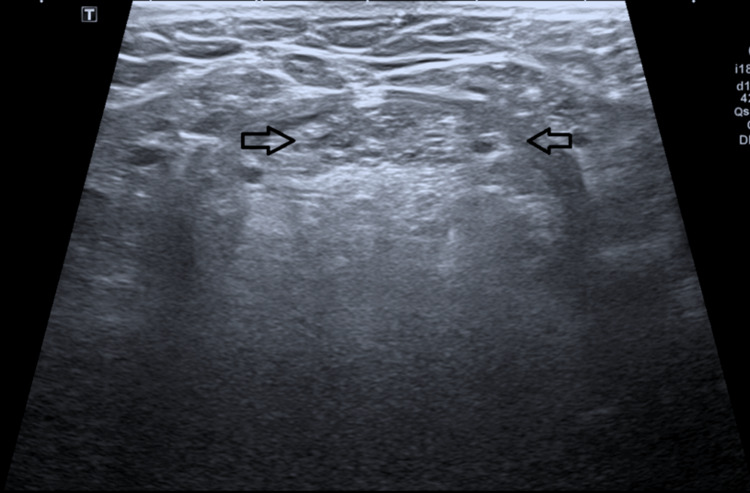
Ultrasound of the thyroid demonstrating mild enlargement, demonstrating inhomogeneous echo pattern with pseudonodular appearance/micronodules and mild increase in vascularity, with features suggestive of chronic autoimmune thyroiditis.
